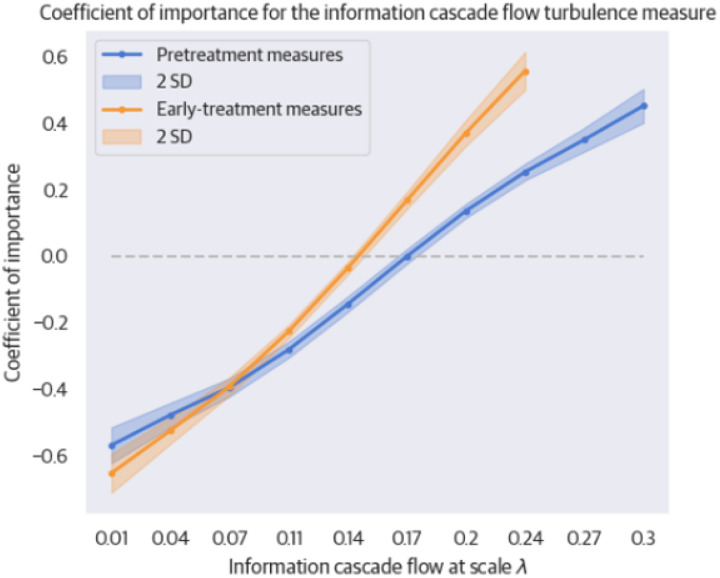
Feature Importance of Information Cascade Flow. The plot shows the coefficient of importance for the information cascade flow turbulence measure from MRI scans acquired pretreatment and one week after treatment initiation (early-treatment). The horizontal axis expresses the scale λ at which the information cascade flow is measured. λ is an inverse distance parameter, such that small values of λ on the left correspond to a larger scale and thus turbulence is measured at greater distances in the brain. The vertical axis shows the coefficient values of feature importance. The magnitude of the value expresses the strength of the feature, whereas the sign expresses its directionality. Thus, high positive values are strongly positively associated with a positive treatment outcome, and low negative values are strongly negatively associated. The magnitude of the coefficient expresses the strength. The shaded regions around each line indicate the variation among models trained in leave-one-out cross-validation, covering two standard deviations. The dashed line at zero serves as a reference point for the coefficients’ significance.
